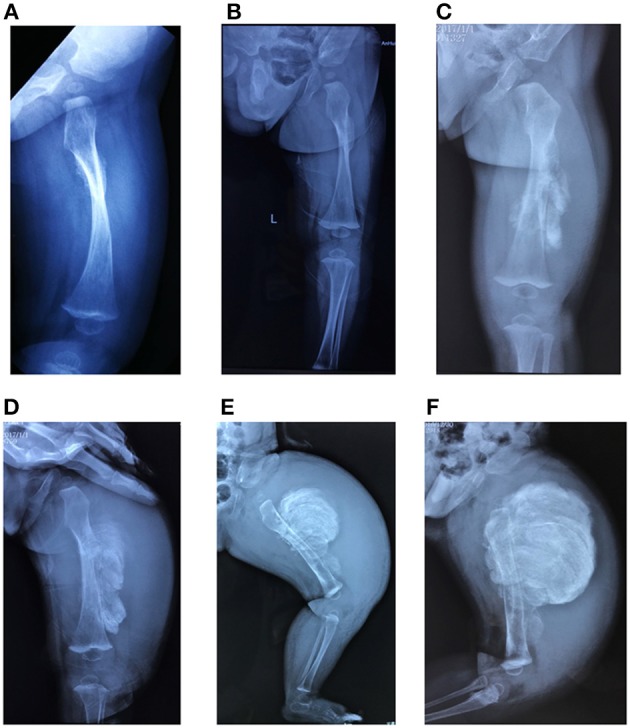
(A–F) Lateral X-rays of the left femur captured 15, 30, 45, 60, 75, and 90 days after fractures. Calluses occurred at the fracture site and increased over time (F3II1). Written informed consent for the publication of this image was obtained.
